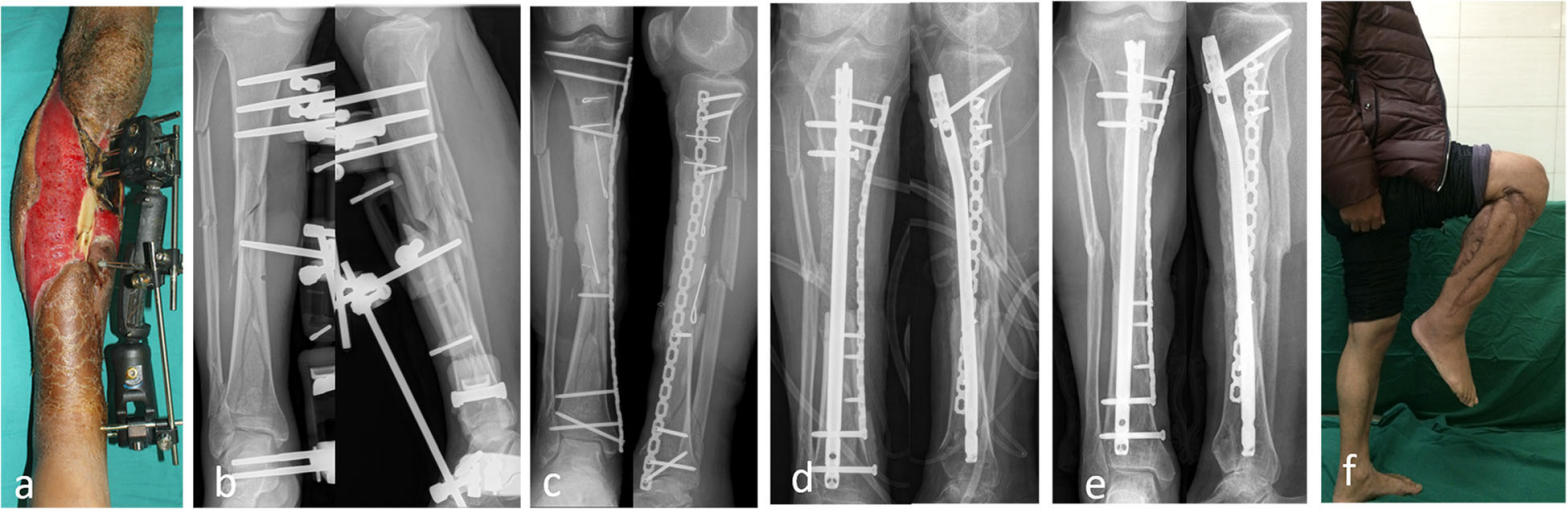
Forty-seven-year-old male. Bone infection after open injury. The culture results showed *Pseudomonas aeruginosa*. a Extensive soft tissue defect and bone exposure. b Preoperative X-ray. c Resection of the lesion. Antibiotic cement filled the defect and wrapped the plate. d Bone graft after 4 months. e Bone union after 24 months. f Function well
